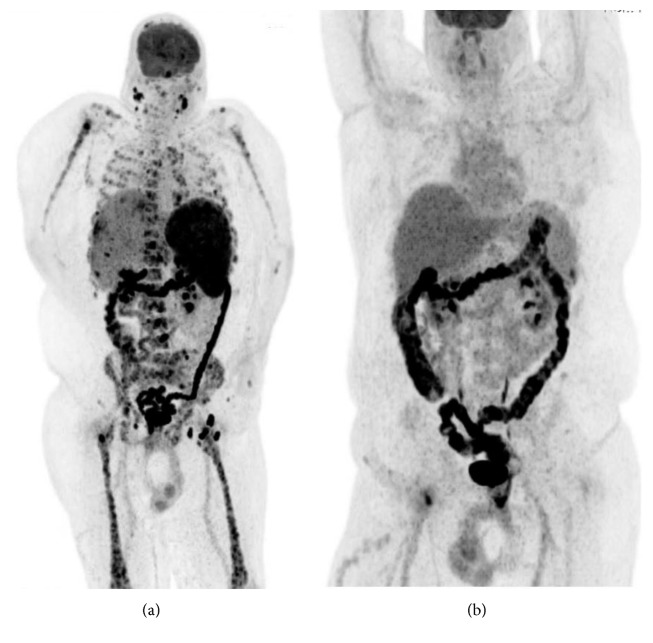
PET-CT images: 3-dimensional MIP images.
